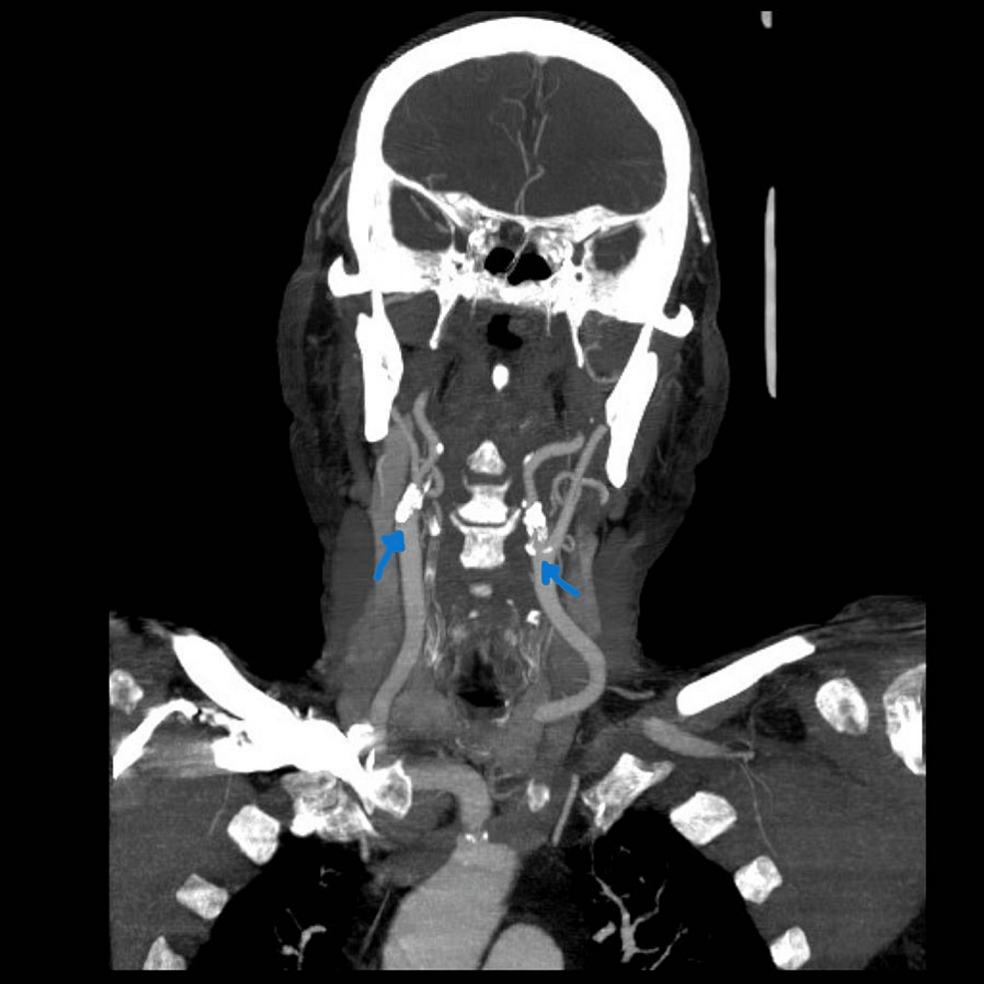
Computed tomography angiography (CTA) Tight stenosis in both internal carotid arteries
